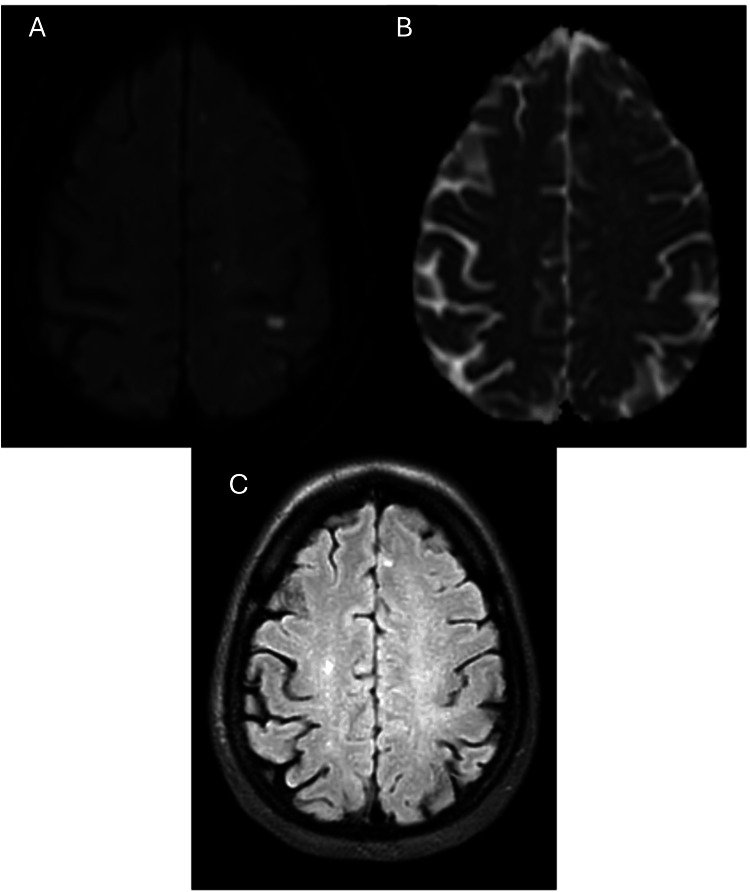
A repeat MRI of the brain revealed: (A) Axial DWI image (b=1000), with corresponding low signal on the axial ADC image (B) demonstrating acute infarct in the left high parietal and centrum semiovale regions. (C) The corresponding axial FLAIR image shows an increased signal at the same region. DWI: Diffusion-weighted imaging; FLAIR: Fluid-attenuated inversion recovery; ADC: Apparent diffusion coefficient
